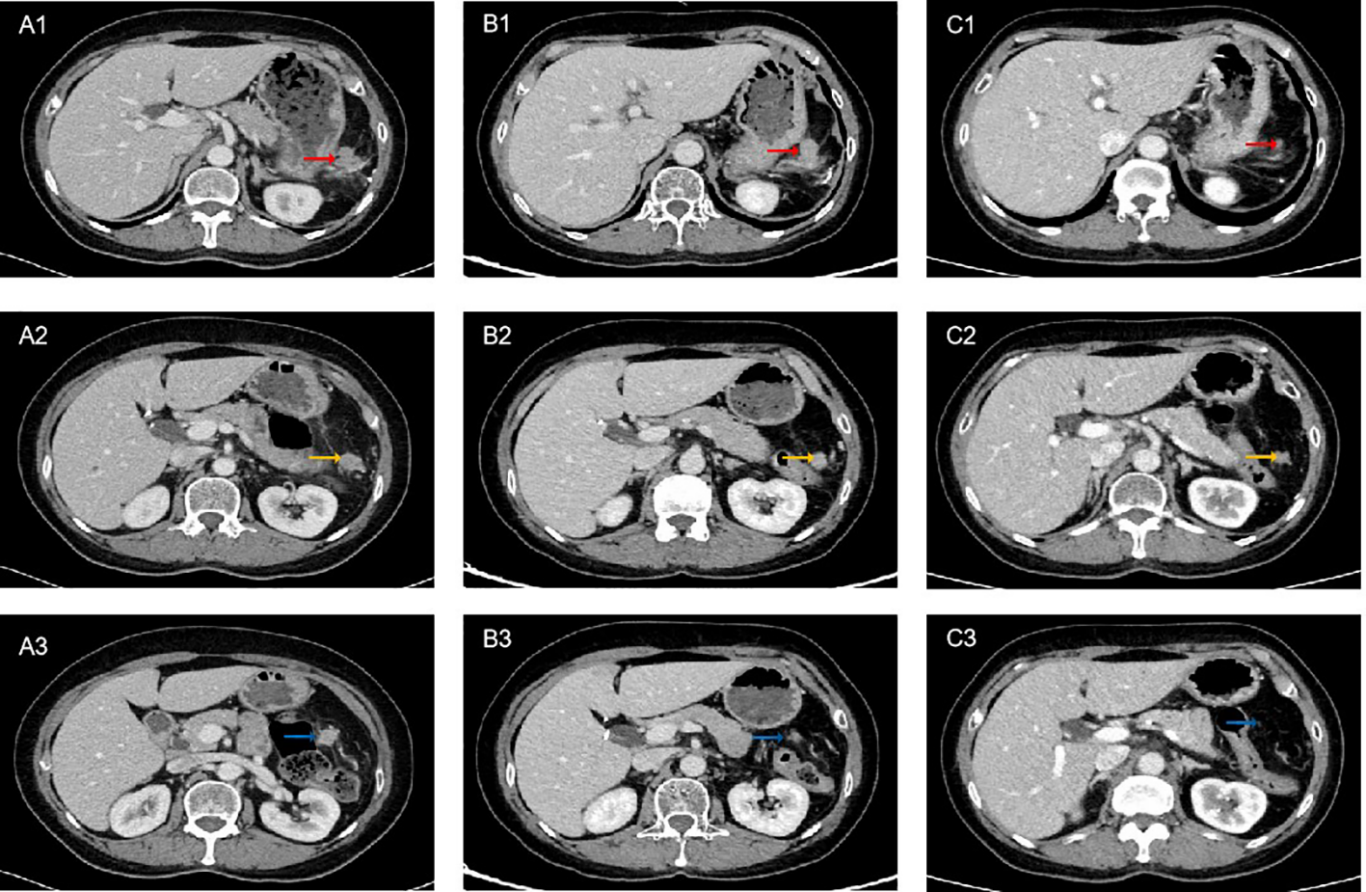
Computed tomography (CT) scan of the patient on treatment. (A1-A3) Pretreatment. (B1-B3) After 5 cycles of PD-1 inhibitor plus chemotherapy, CT showed partial response (PR). (C1-C3) After 8 cycles of PD-1 inhibitor plus chemotherapy combined with radiotherapy, CT showed sustained PR. The red arrows indicate lesion 1, yellow arrows indicate lesion 2, and blue arrows indicate lesion 3.
